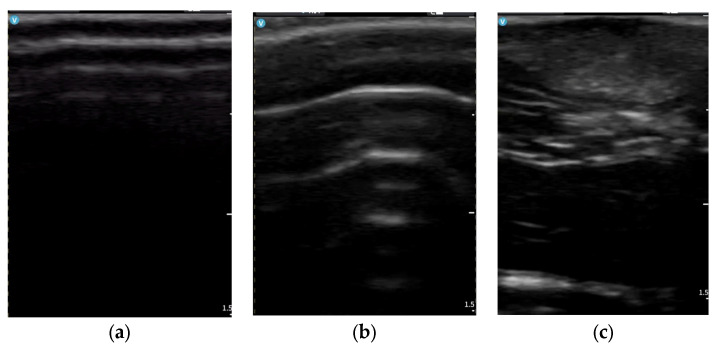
Representative longitudinal ultrasonographic images of the same anatomical region across timepoints. (a) Baseline (Day 0): preserved dermal–subcutaneous architecture with a homogeneous hypoechoic subcutaneous layer and fine echogenic septa. (b) Day 17: increased echogenicity, septal thickening, and reduced structural homogeneity consistent with early fibrotic remodeling. (c) Day 31: partial attenuation of echogenicity and septal prominence with the presence of focal hypoechoic areas corresponding to graft integration.
